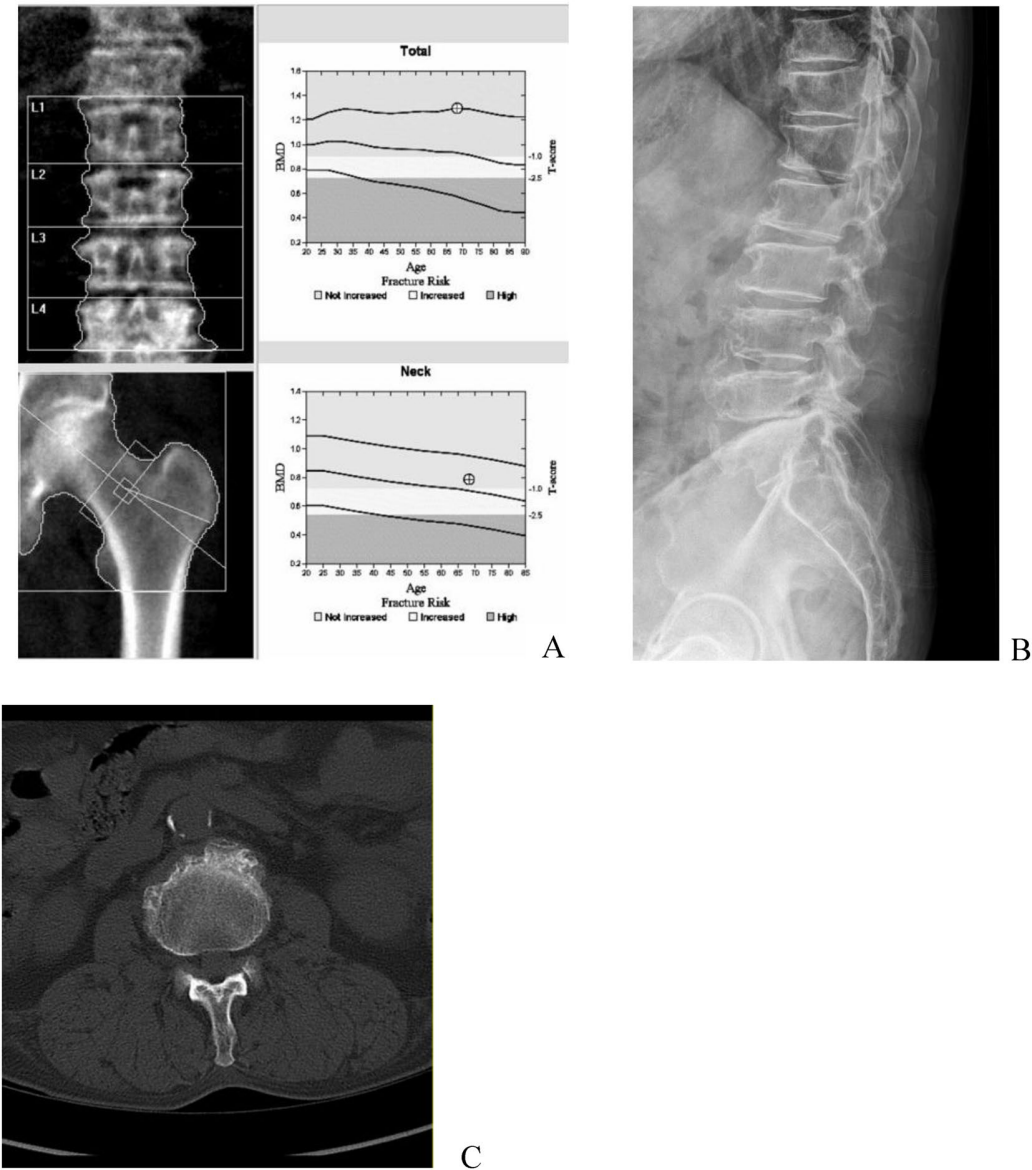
Images obtained from a 68-year-old man who was diagnosed via DXA to have normal CT scans (A–C). (A) T-scores for lumbar DXA, femoral neck, and total hip were 1.4, − 0.5, and − 0.5, respectively. The trabecular BMD of L1-L2 was 77.125 mg/cm^3^, showing a diagnosis of osteoporosis according to the American College of Radiology (ACR) guidelines. A lateral lumbar spine radiograph (B) and an axial CT image (C) depicted severe osteophytes and end plate sclerosis of the lumbar vertebrae. Abdominal aortic calcifications also were noted.
